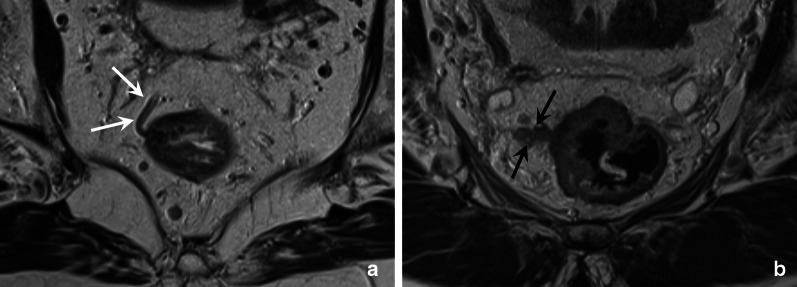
Axial T2-weighted images of two rectal cancer patients; a 63-year-old male patient with a cT1-2 rectal tumour (a; tumour not shown) an another 83-year-old female patient with a cT3cd tumour (b). The image in A is a cross section just above the level of the tumour in the rectal wall and shows a small vessel radiating outwards from the muscularis propria into the perirectal fat (white arrows). The vessel has a normal contour and low T2 signal; there are no signs of EMVI. The image in b shows a semicircular tumour that involves the rectal wall from 7 till 1 o’clock. The intermediate signal of the tumour extends into the adjacent vessels. The vessels is expanded, and the vessel contour is disrupted. These are all signs indicative of EMVI
